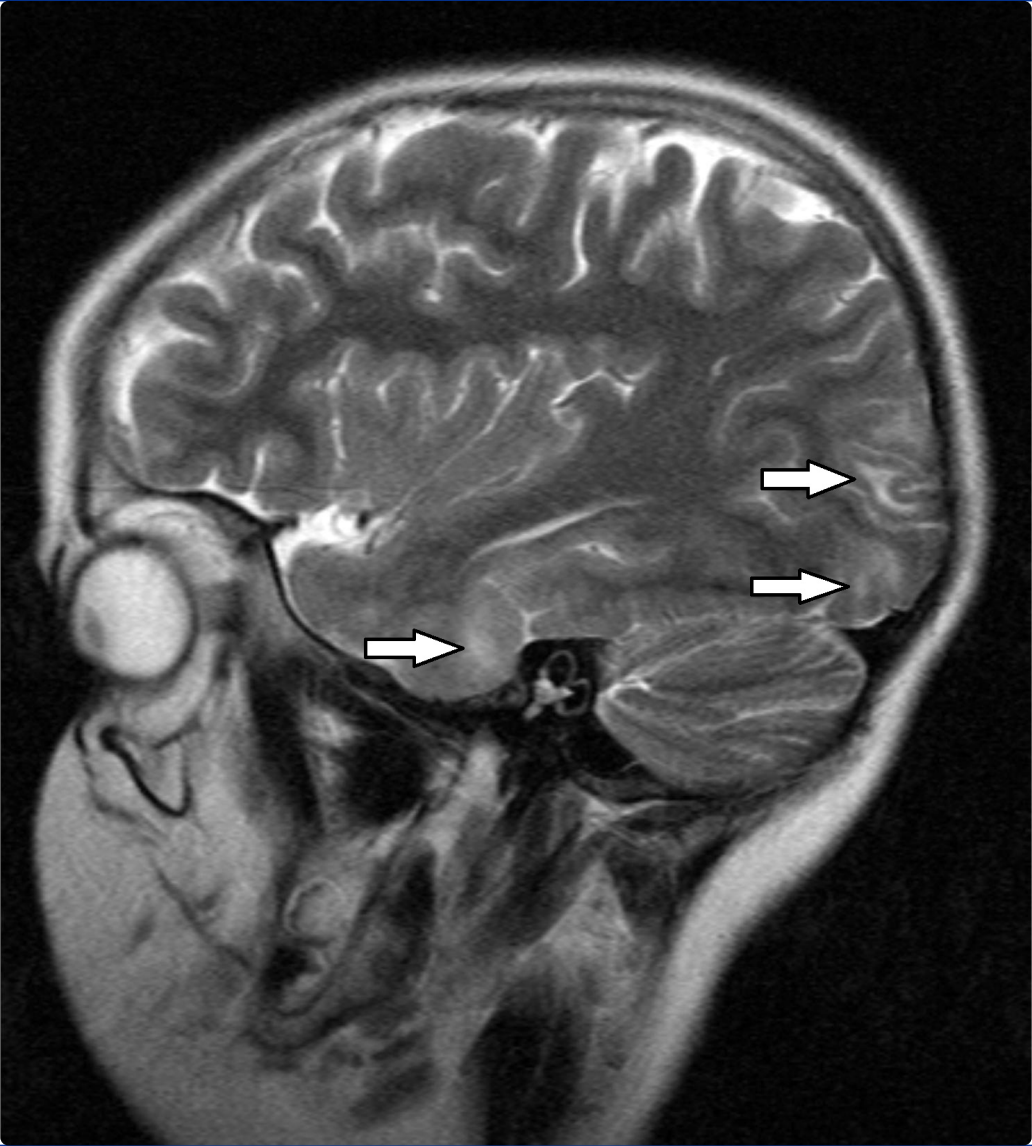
T2 sagittal MRI through a right paracentral position showing multiple subcortical white matter lesions in the right temporal lobe anteriorly, and the right occipital lobe posteriorly.
